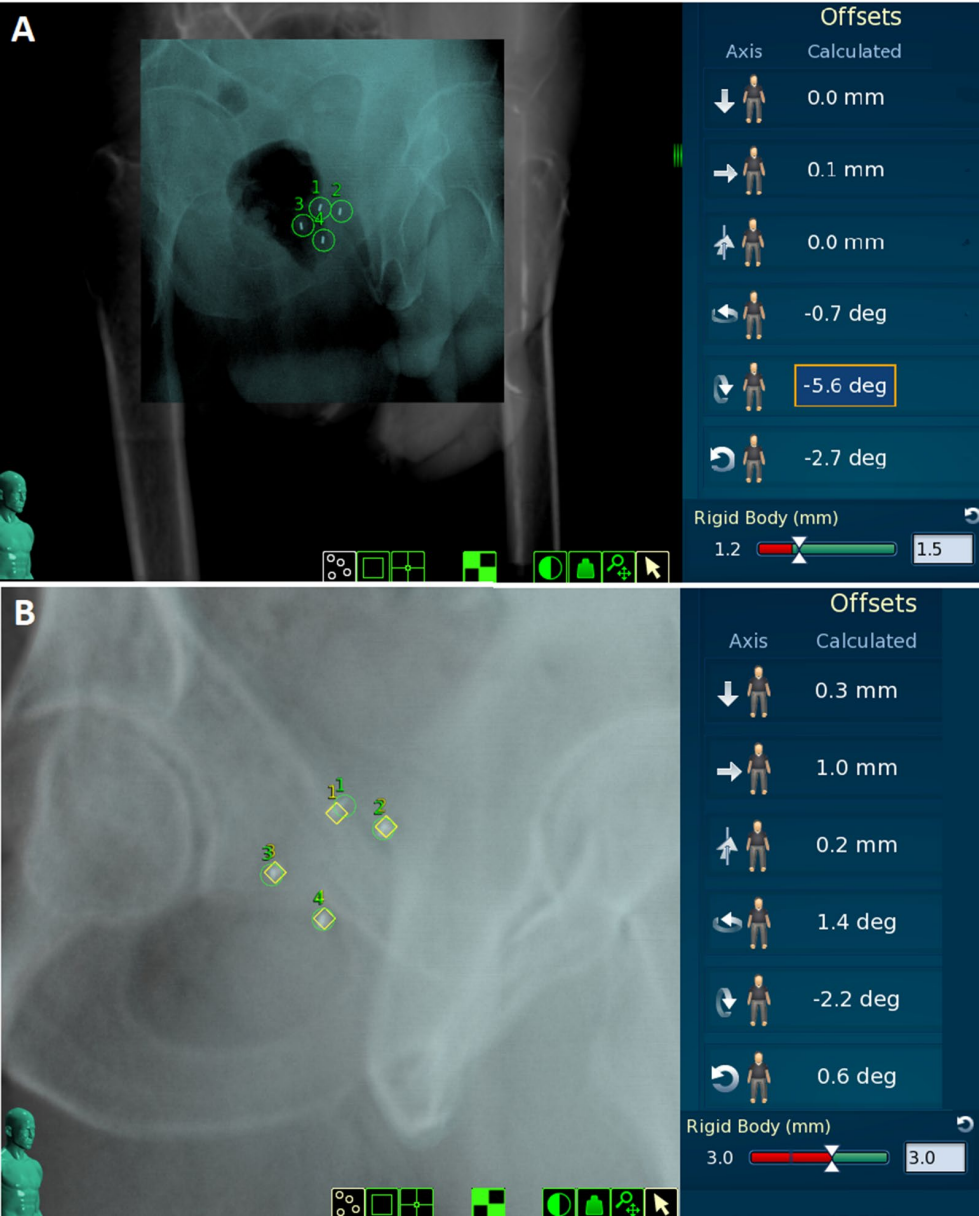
Impact of rectal and bladder filling on prostate position and deformation. Panel a shows gas in the rectum causing high-pitch rotation and interruption of the treatment while the RBE is below threshold. Panel b shows a comparison of 4 FMs’ positions between the reference CT (rhombus) and during treatment (circle), with the translational and rotational offsets and the highest detected rigid body error (in FM1)
